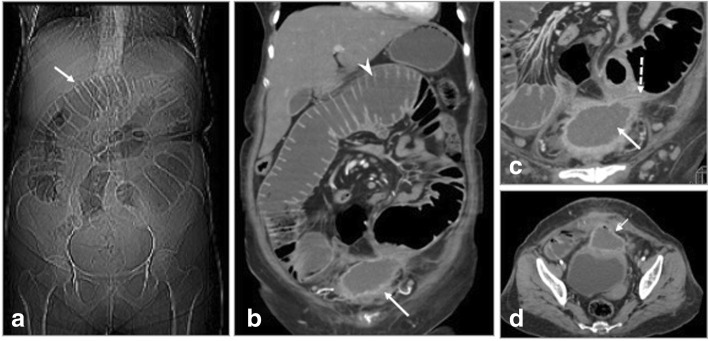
Multiple complications in a post ventral hernia repair. a CT scanogram shows signs of small bowel obstruction with severely dilated loops (arrow). b, d Coronal and axial CECT confirm the same (arrowhead) with collection suggesting mesh migration (arrow). c Magnified view of coronal section shows fistulization (dashed arrow) with small bowel
